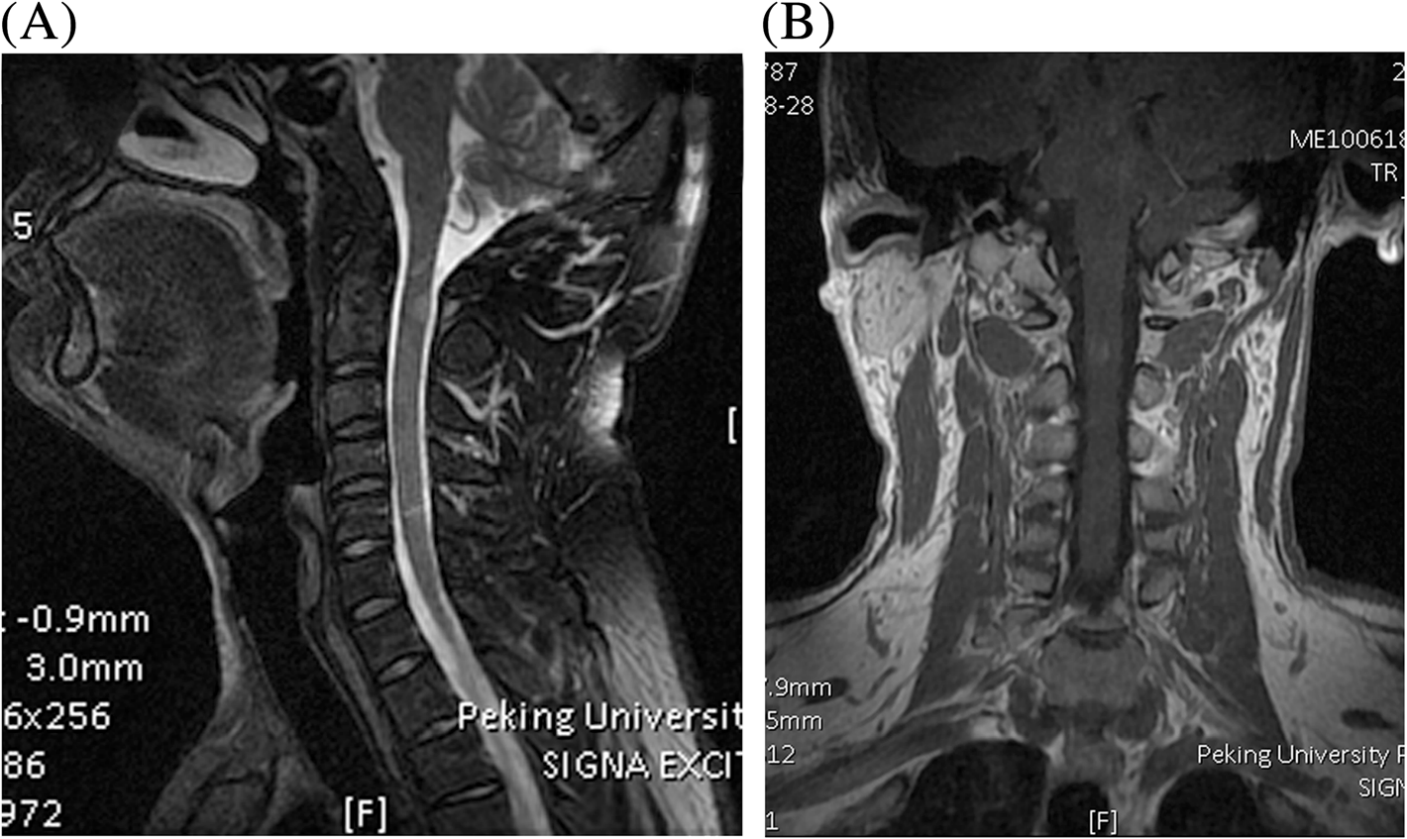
Magnetic resonance imaging (MRI) scans from a 29-year-old male patient who presented with a five-week history of numbness and uncoordinated right upper limb activity for one week. The patient had a nodular cervical spinal cord lesion. (A) T2-weighted imaging showed hyperintense and (B) enhanced MRI images identified uniform nodular lesions.
